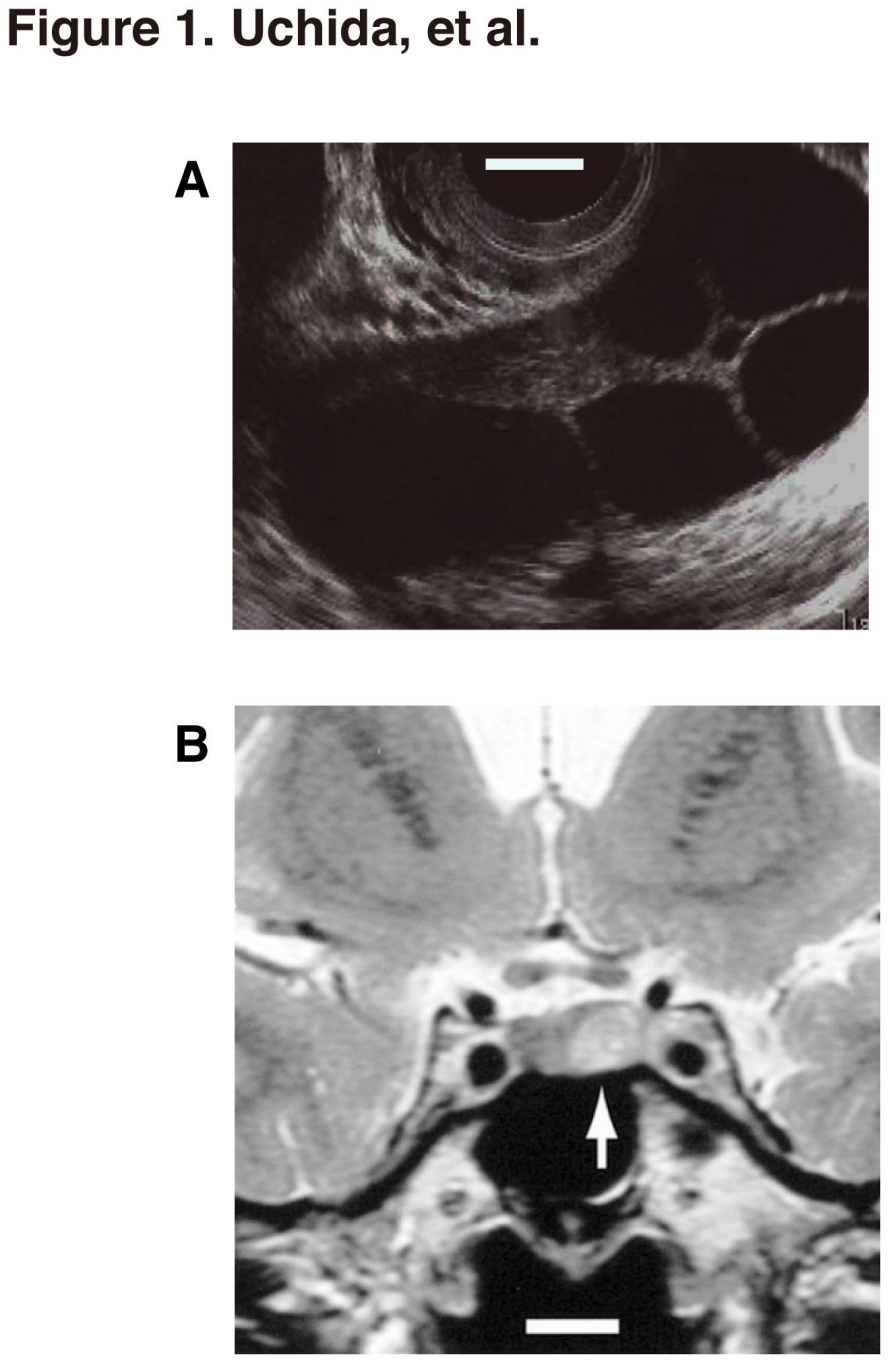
Multiple ovarian cysts and the pituitary microadenoma in the patient. (A) Transvaginal ultrasonographs of the right ovary taken at the initial visit. Bar, 1 cm. (B) T2-weighted coronal magnetic resonance imaging performed 3 months after the initial visit revealing an intra-sellar tumor measuring 9 mm diameter, with a high signal intensity in the left side of the pituitary (arrow). Bar, 1 cm.
